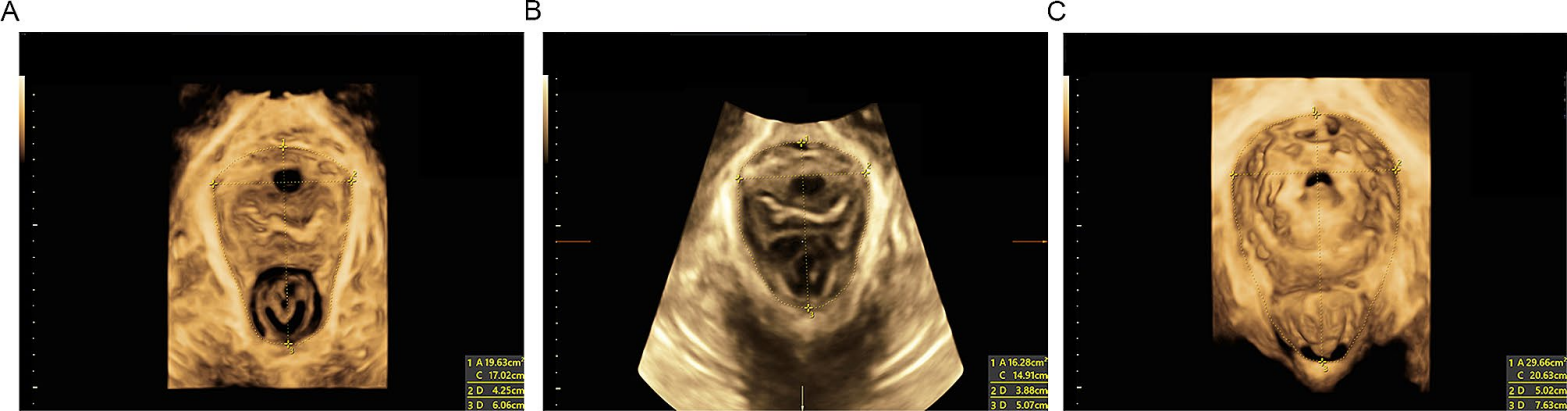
Four-dimensional hiatus sonography (1 A: basin septal hole area; 1 C: basin septal hole circumference; 2: basin septal hole diameter; 3: anterior and posterior diameters of basin septa). (A) Resting state. (B) Retracted anus state. (C) Maximum Valsalva state
